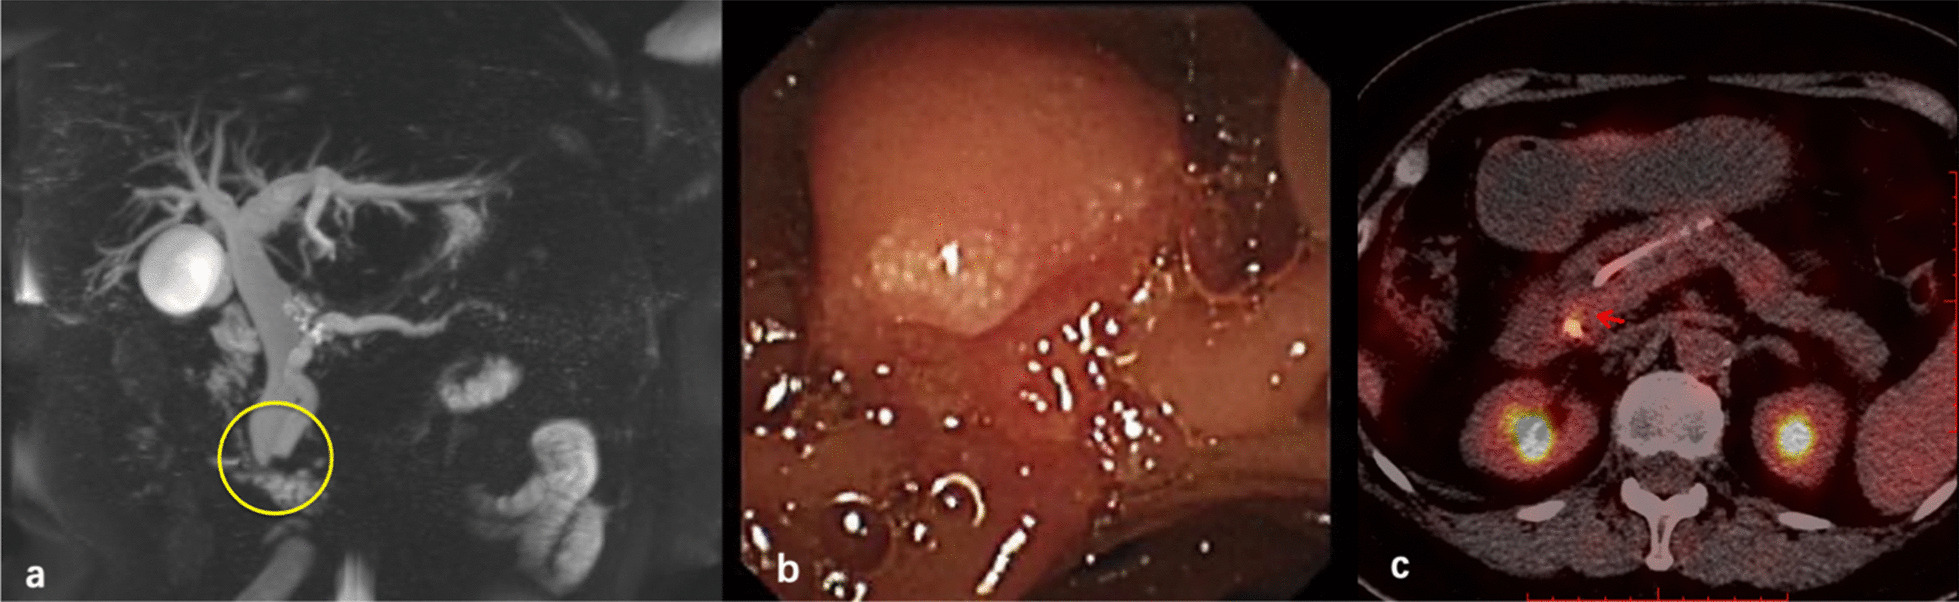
MRCP suggested the common bile duct and main pancreatic duct were suddenly narrowed at the duodenal ampulla (a). ERCP showed a bulging protrusion at the ampulla of Vater (b). PET/CT revealed an increased metabolic focus at the duodenal papilla area (c)
